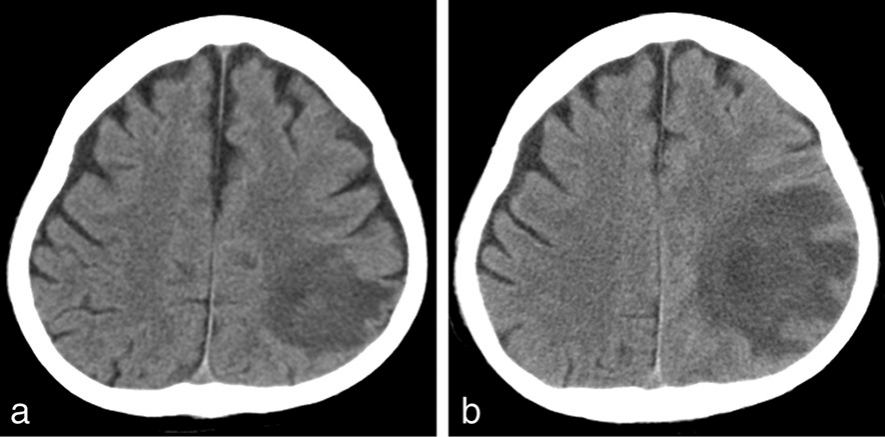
(a) Brain CT image demonstrating a low density mass in the left parietal lobe. (b) Brain CT image taken 2 days later demonstrating rapid growth of the low density mass.
